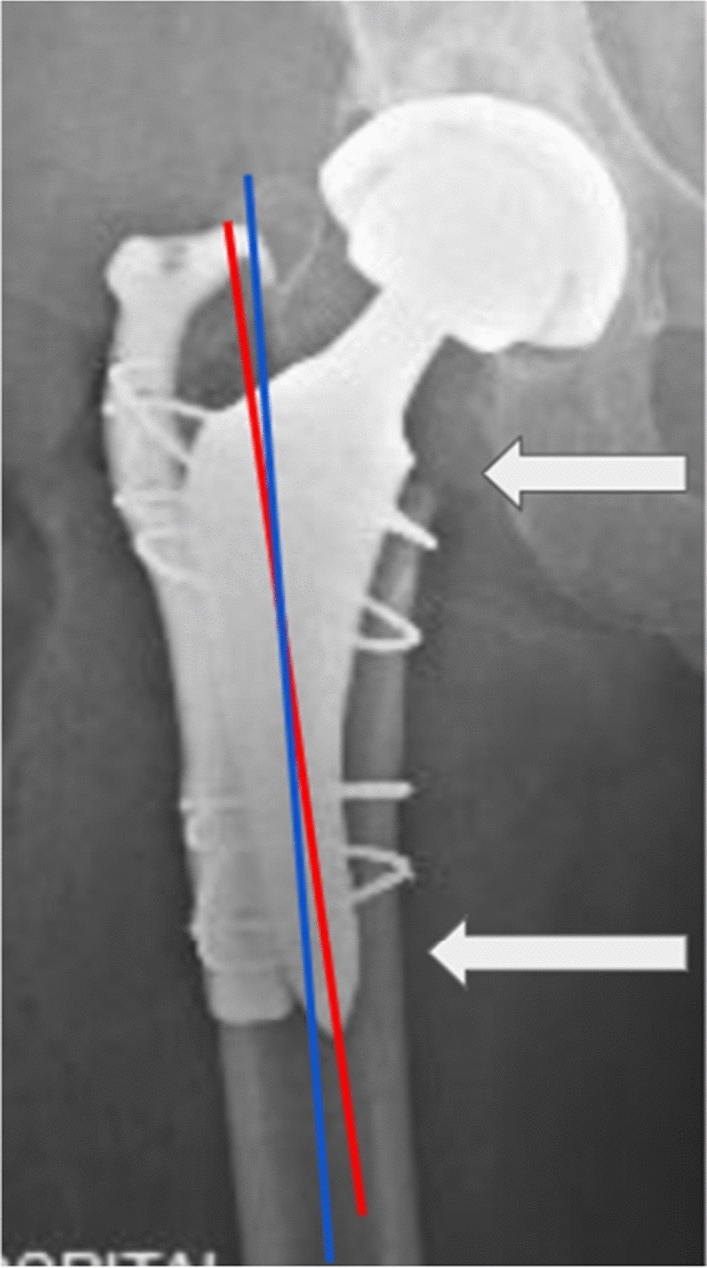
AP radiograph of the right hip resulted in an intraoperative displaced greater trochanter fracture managed with a proximal femur plate and cables. The stem is in significant valgus in relation to the femoral intramedullary axis
